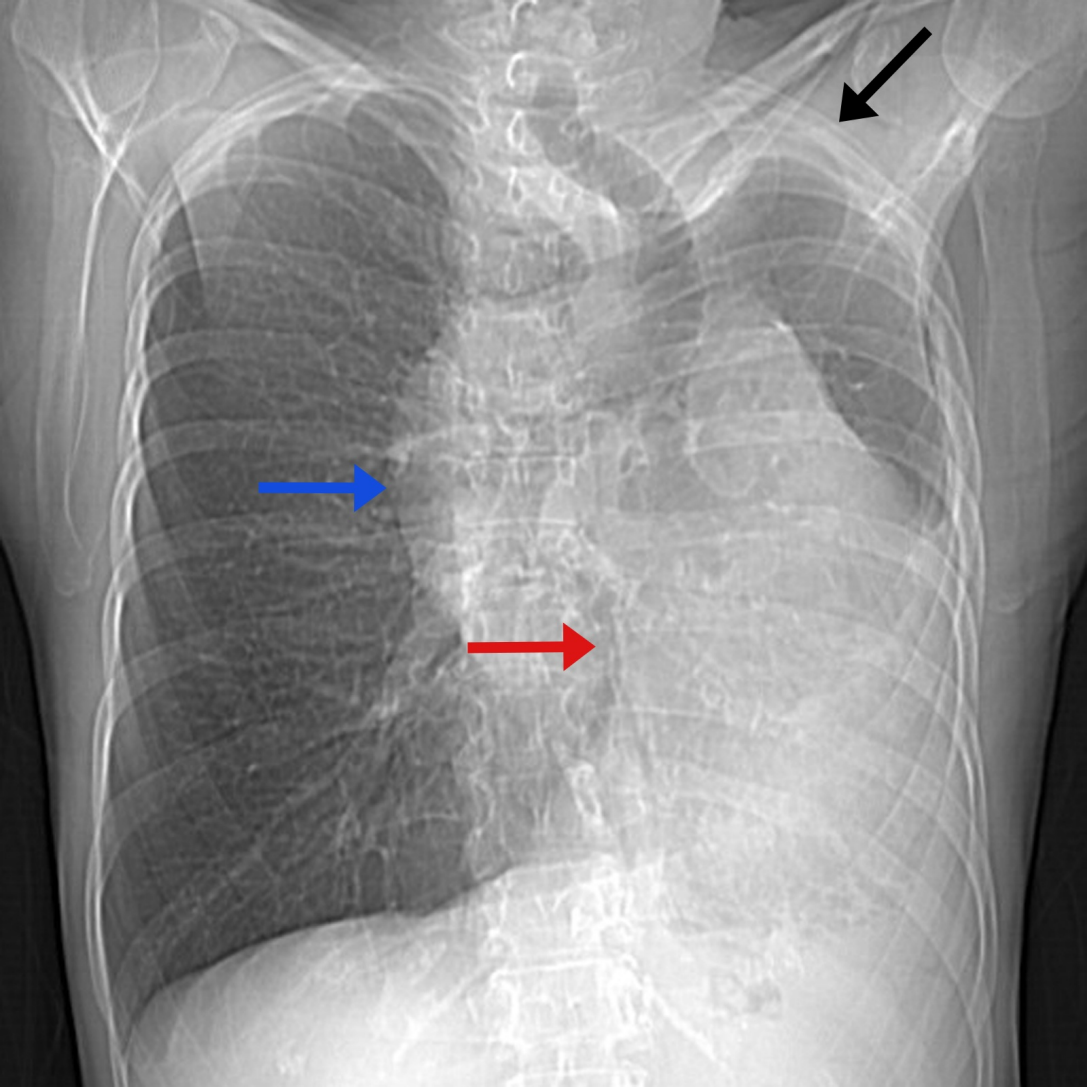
CT chest, scout image. There is leftward shift of the cardiomediastinal silhouette (red arrow) with hyperinflation of the right lung; no evidence of left lobe collapse. The left hemithorax is asymmetrically smaller when compared to the right (black arrow). There is central left-sided bronchiectasis with opacification of the left lower lung field. Incidental note is made of a right-sided aortic arch (blue arrow).
